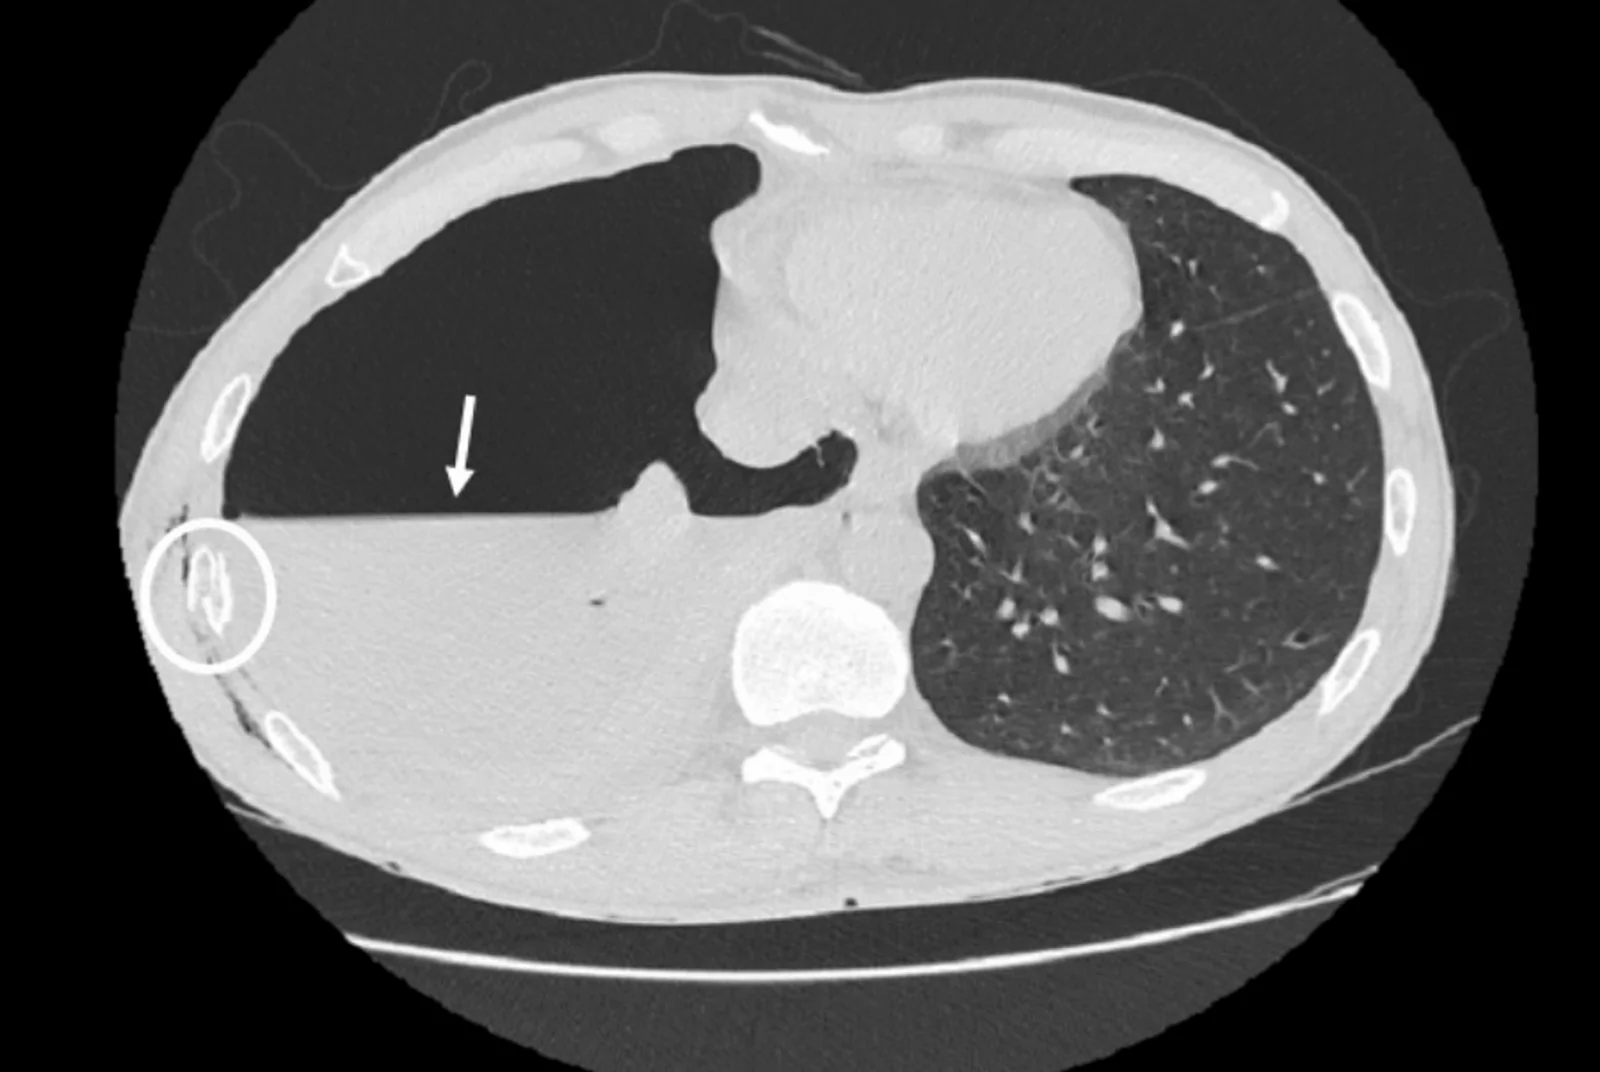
Axial computed tomography scan demonstrating a displaced rib fracture (lung window), taken on day one of admission. The scan reveals a displaced lateral right-sided rib fracture of the eighth rib (white circle) accompanied by a right-sided hydropneumothorax. The white arrow marks the flat air-fluid level.
